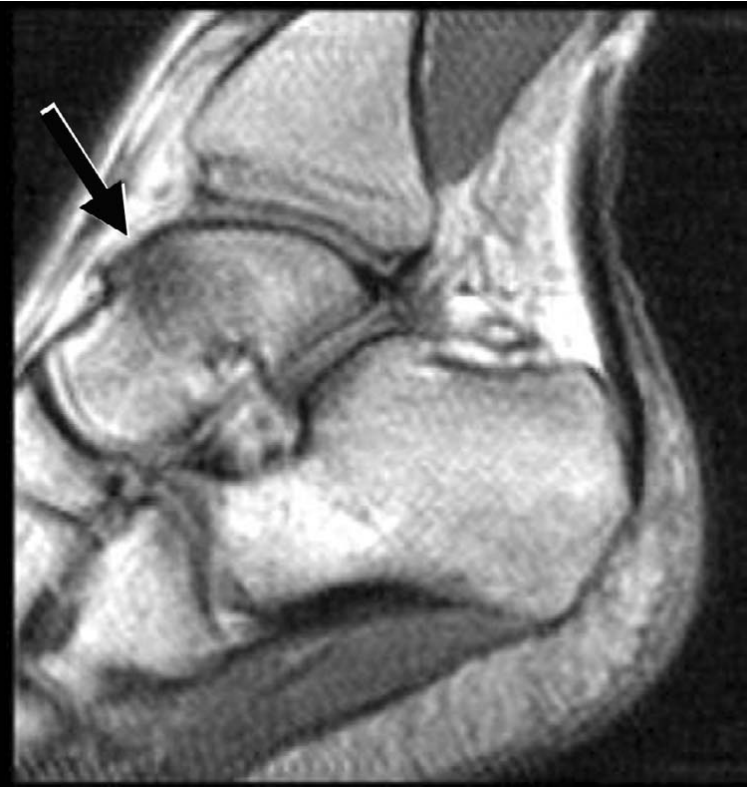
Talar bone marrow edema in a symptomatic 24-year-old male (same subject as in figure 1). Sagittal T1-weighted (TR/TE = 380/18) MR image shows patchy low signal within the talus (arrow).
